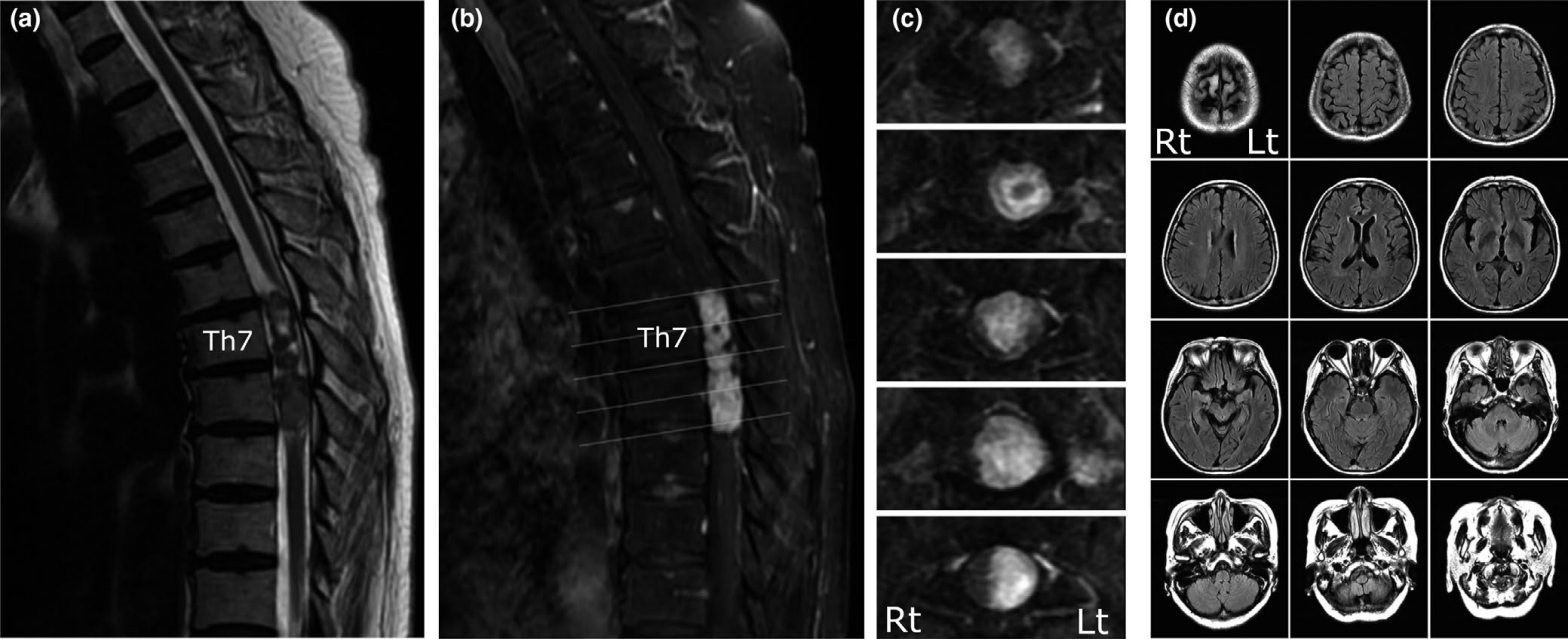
Spinal cord and brain MRI. MRI demonstrated the unilateral spinal cord tumor and retrograde regression of the corticospinal tract (CST) fibers. T2‐weighted images showed mixed intensity signal mass in the thoracic spinal canal at the T7 and T8 levels (a). T1‐weighted gadolinium‐enhanced images showed an enhanced intradural extramedullary mass which was located on the left side of the spinal cord and displaced the spinal cord to the right (b, c). Fluid‐attenuated inversion recovery images in the brain seemed normal (d)
